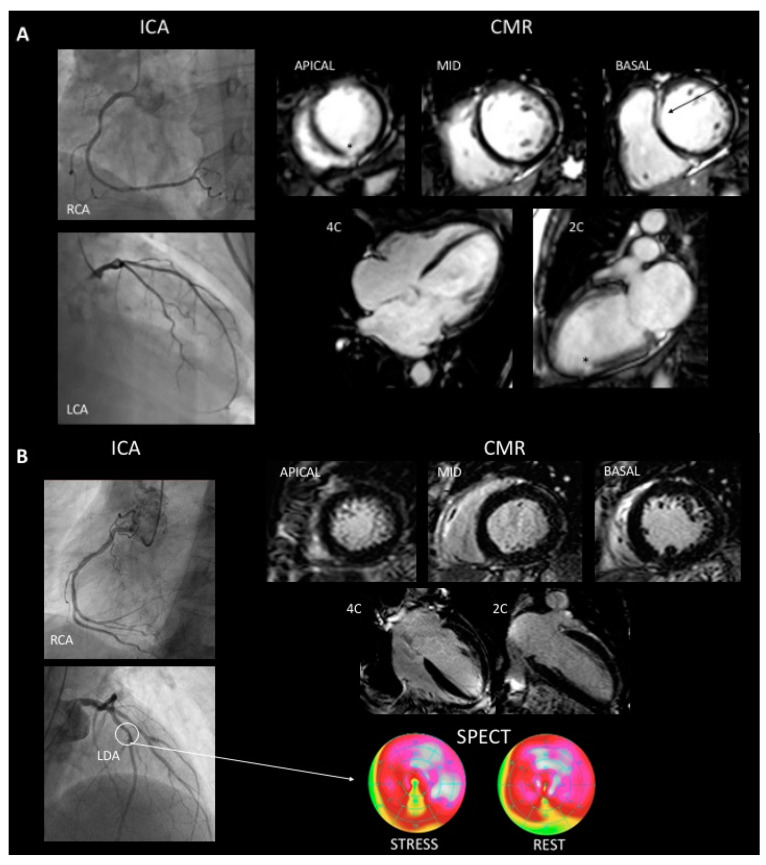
A 66-year-old woman who presented withg dCMP and bystander myocardial infarction. ICA was normal and CMR showed a 31% LVEF, an end-diastolic volume of 131 mL/m^2^, global hypokinesia and 2 patterns of LGE. Mid-wall linear septal (arrow) and small sub-endocardial LGE (asterisk) (Panel A). A 71-year-old man who presented dCMP with coronary artery disease. ICA shows plaque to 40–50% on left descending anterior artery (arrow), CMR showed a 32% LVEF, an end-diastolic volume of 123 mL/m^2^, global hypokinesia and no LGE (Panel B). LCA: Left coronary artery, LDA: left descending artery, RCA: right coronary artery, ICA: invasive coronary angiogram, CMR: cardiac magnetic resonance imaging, SPECT: single photon emission computed tomography.
